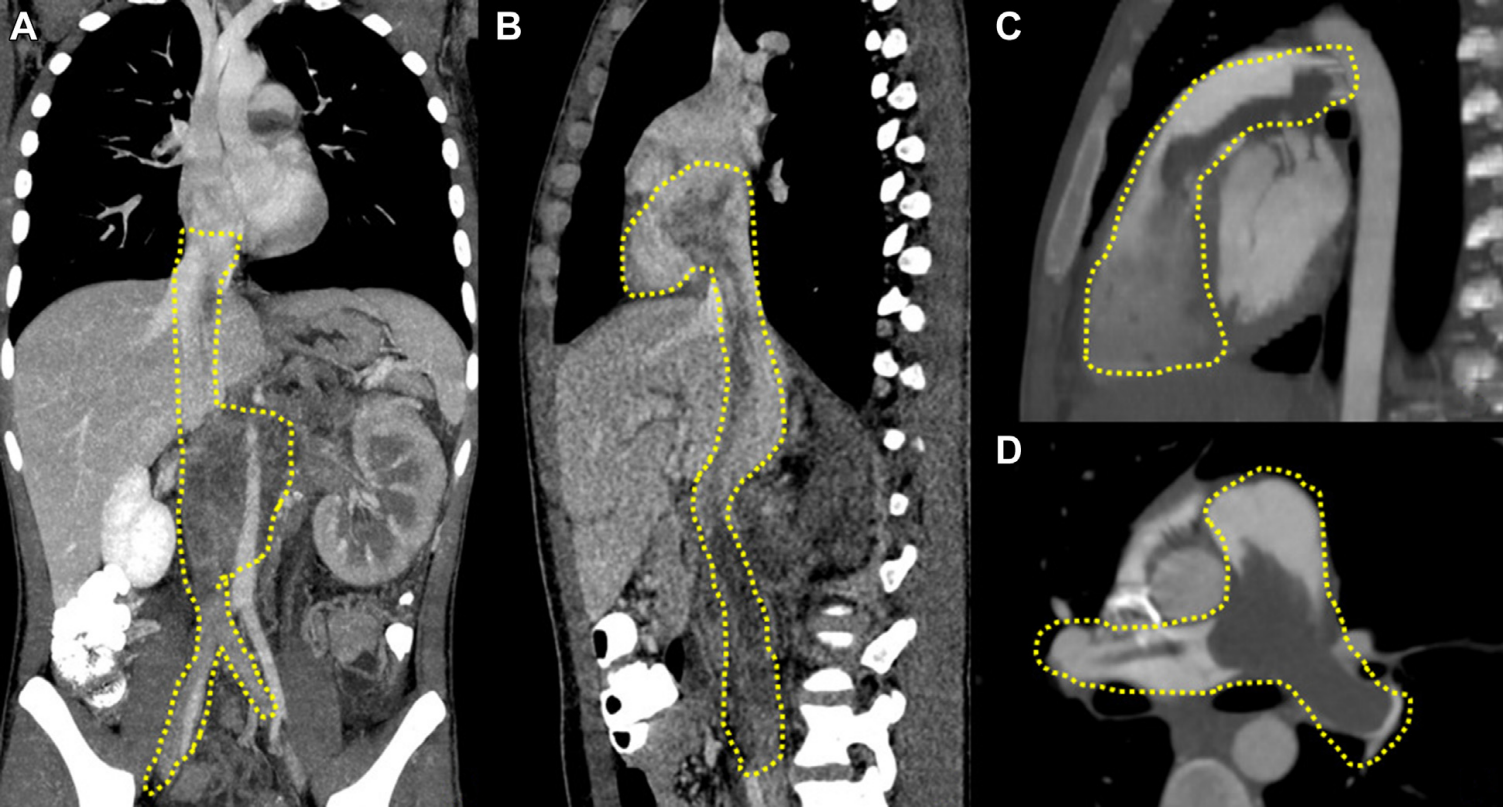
Thoracoabdominal CT Angiography The yellow dotted lines outline the vascular structures. (A) Coronal plane. Retroperitoneal tumor infiltrating the inferior vena cava and extending to the intrahepatic portion of the vena cava. (B) Oblique sagittal reconstruction. Extension of the tumor toward the right atrium. (C) Oblique sagittal reconstruction. Extension of the tumor toward the right ventricle and pulmonary trunk. (D) Axial plane. Extension of the tumor toward the pulmonary arteries. CT = computed tomography.
